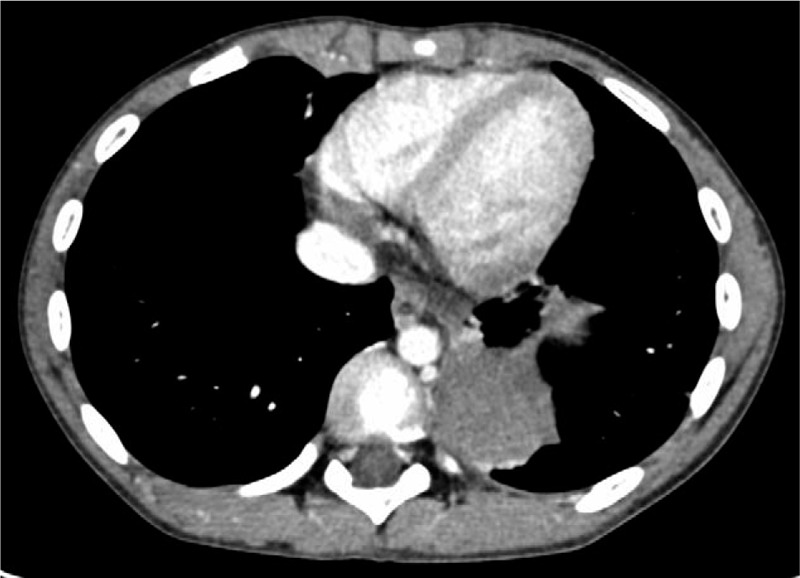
Torsion of extralobar pulmonary sequestration in a 10-year-old boy. Contrast medium-enhanced chest computed tomography showed a heterogeneous solid mass with no enhancement in the left lower thorax.
